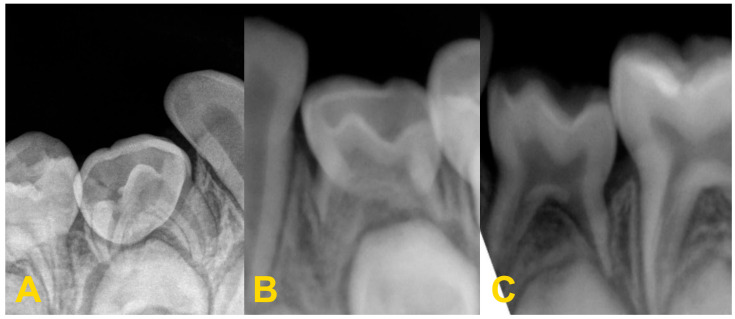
Radiological imaging—dental X-ray of teeth nos.: (A) 84, (B) 74, and (C) 74.
